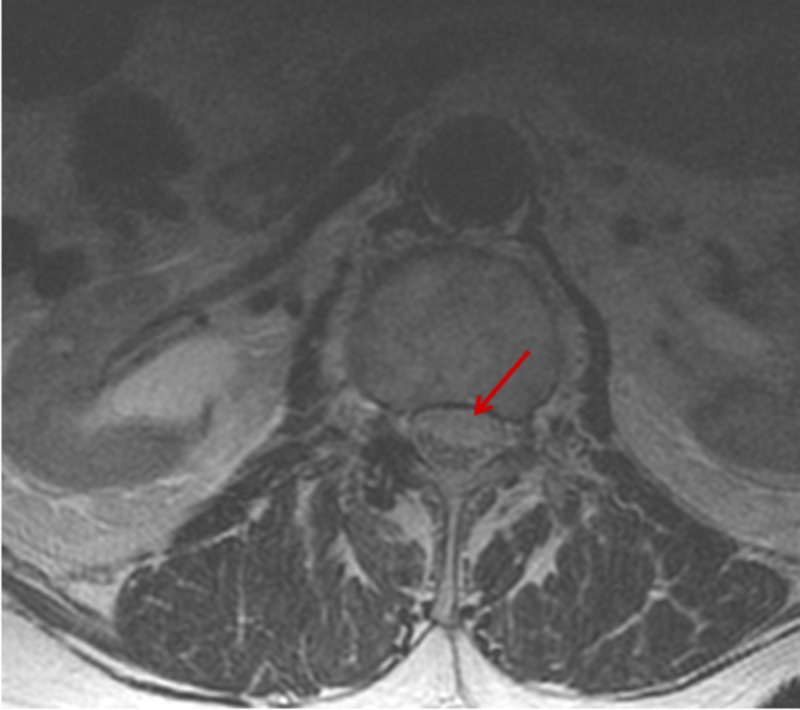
MRI scan, T2-weighted axial view of the lumbar spine demonstrates subdural intraspinal subdural hematoma with mild ventral mass effect on the cauda equina nerve roots (red arrow)
